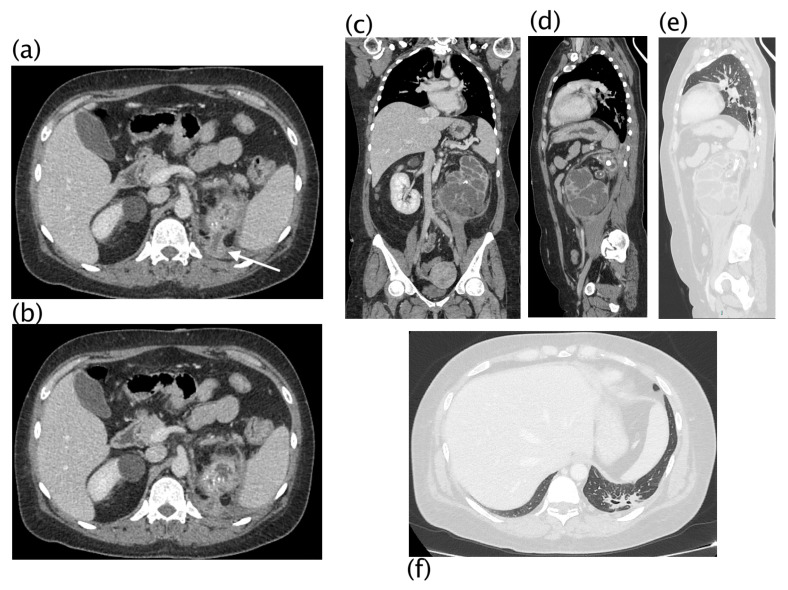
Xanthogranulomatous pyelonephritis Stage III—pararenal form CT with contrast agent: axial (a,b,e), coronal (c), and sagittal (d) MPR reconstructions with lung window (e,f). Patient admitted for persistent cough and left basal opacity on chest X-ray examination. On CT examination, morpho-structural alteration of the kidney with gross intraparenchymal collections alternating with dilated calyces is present. An upper polar fistulous via (a,b) is well evident (white arrow) that continues into the lung in direct continuity with a bronchus, more evident in sagittal reconstructions. Recognition of the fistulous via on CT was visible in 22.22% of patients.
